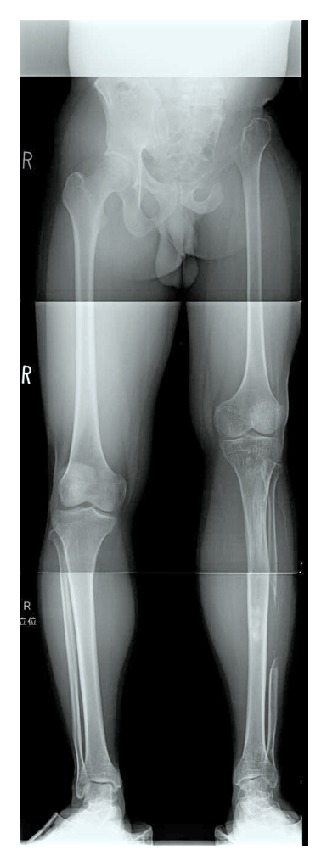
Radiograph of entire lower limbs at 5 years after leg lengthening.
